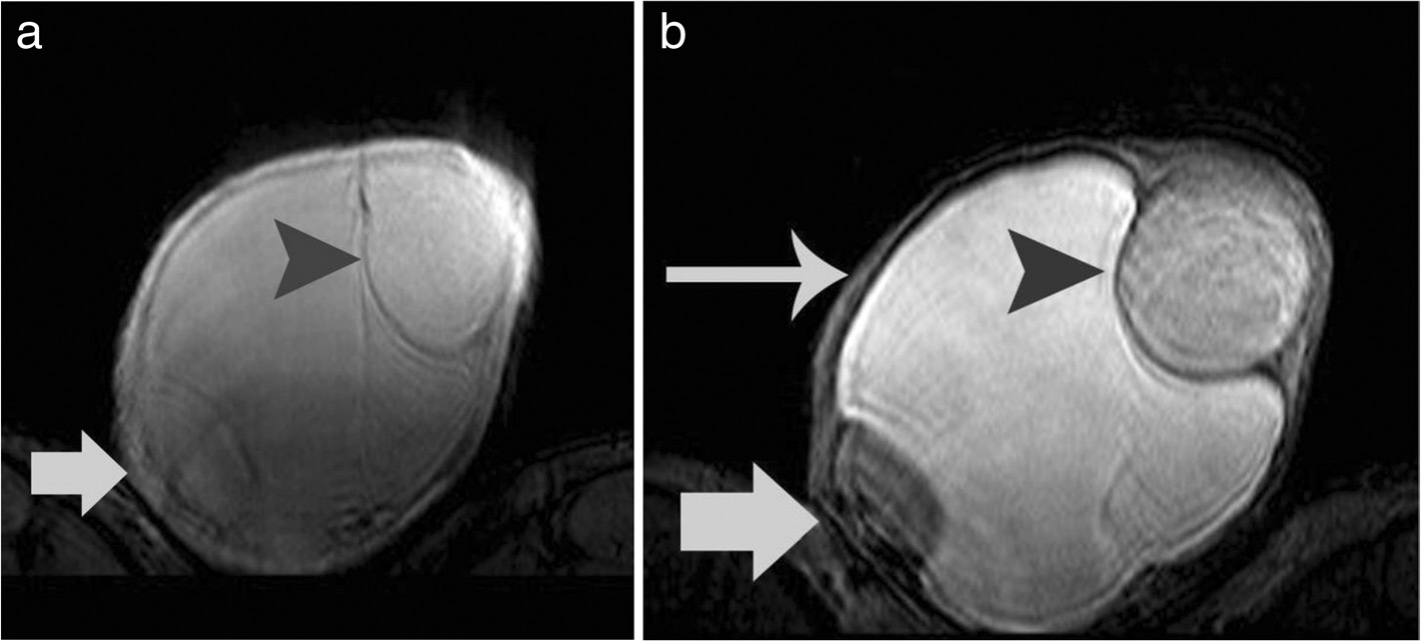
Axial three-dimensional gradient-echo magnetic resonance image before (a) and after (b) the application of the magnetization transfer prepulse. The magnetization transfer ratio (in percent) of the left paratesticular mass (*arrowhead*) was 44.6, similar to that of the contralateral normal testis (46 %, not shown on images). The left testis (*arrow*) was displaced and compressed and the measurement of the magnetization transfer ratio was impossible due to artifacts. Left hydrocele (*long arrow*)
